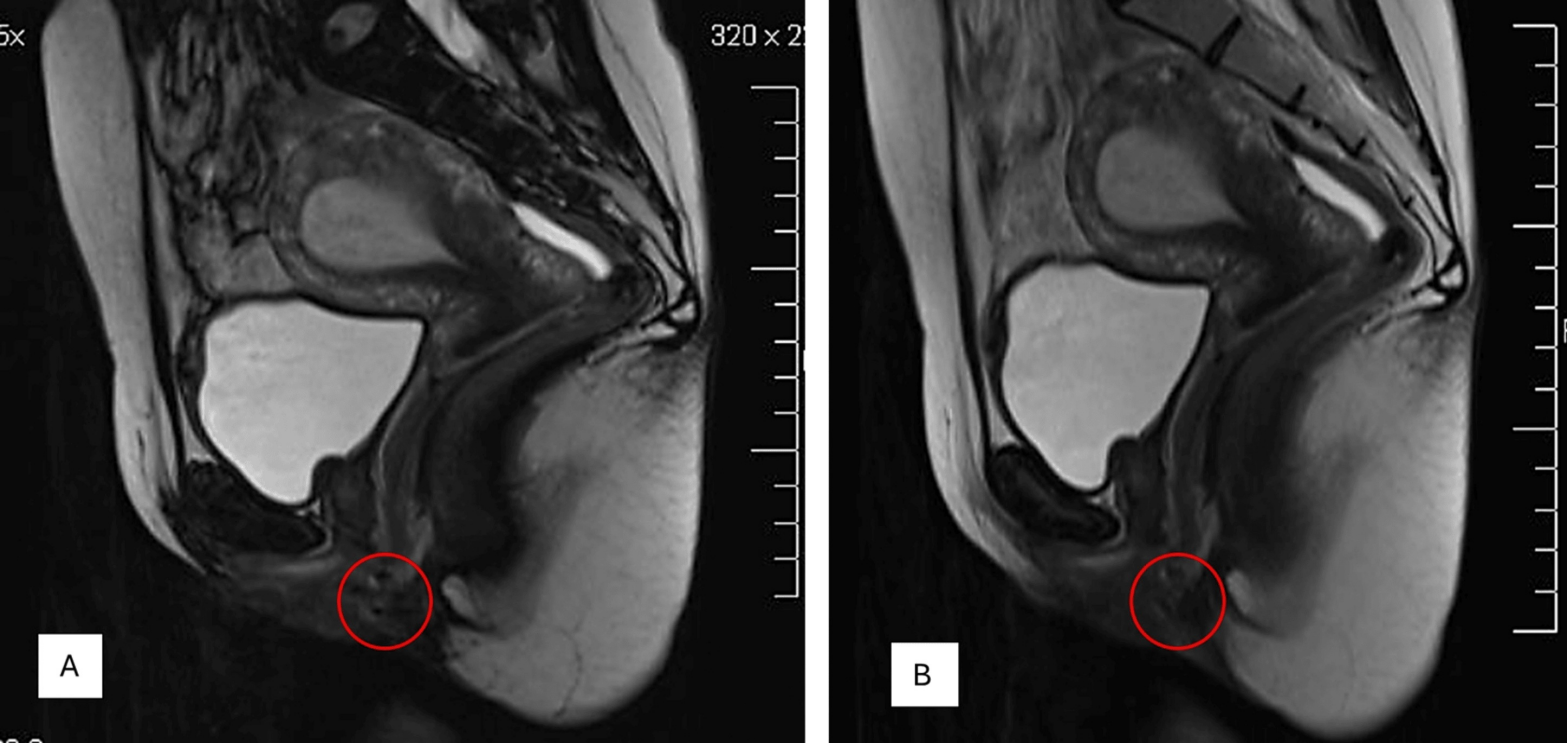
(A and B) Magnetic resonance image (MRI) showing a small lower vaginal and para-urethral hypodense lesion. Red circles highlight the lesion.
